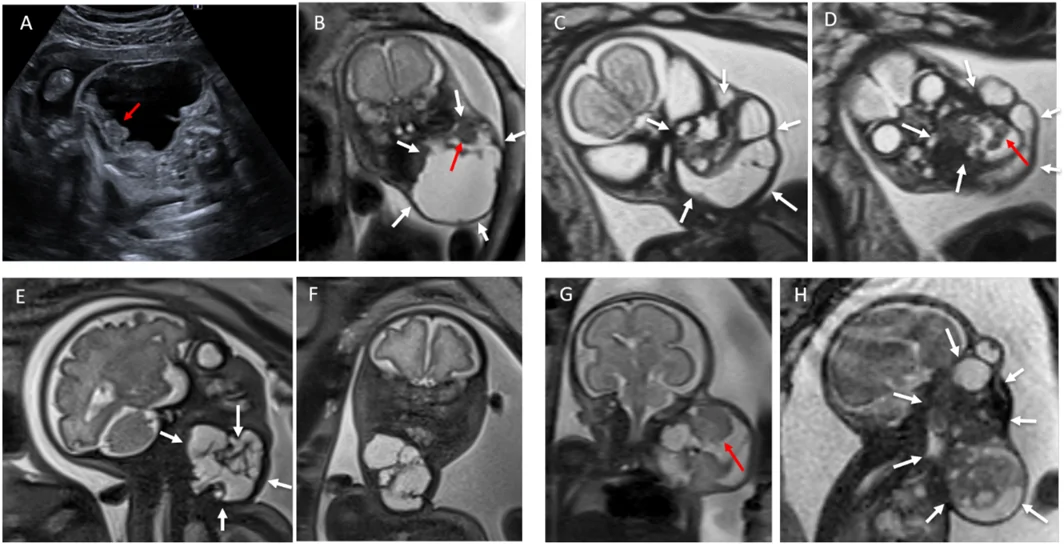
(A‐H): Prenatal imaging of four of our 15 patients referred for extensive cevico‐facial cystic lesion suggestive of LM, in whom review of the ultrasound and fetal MRI examinations raised suspicion of teratoma. Review of the prenatal imaging demonstrated a well circumscribed lesion (small white arrows) with intracystic solid vegetation (red arrow) with major mark effect of this orbite for Patient 4 (H). Patient 1: A,B, Patient 2: C,D, Patient 3: E,F, Patient 4: G,H. In all cases, diagnosis of teratoma was confirmed either pathologically or post‐natally.
